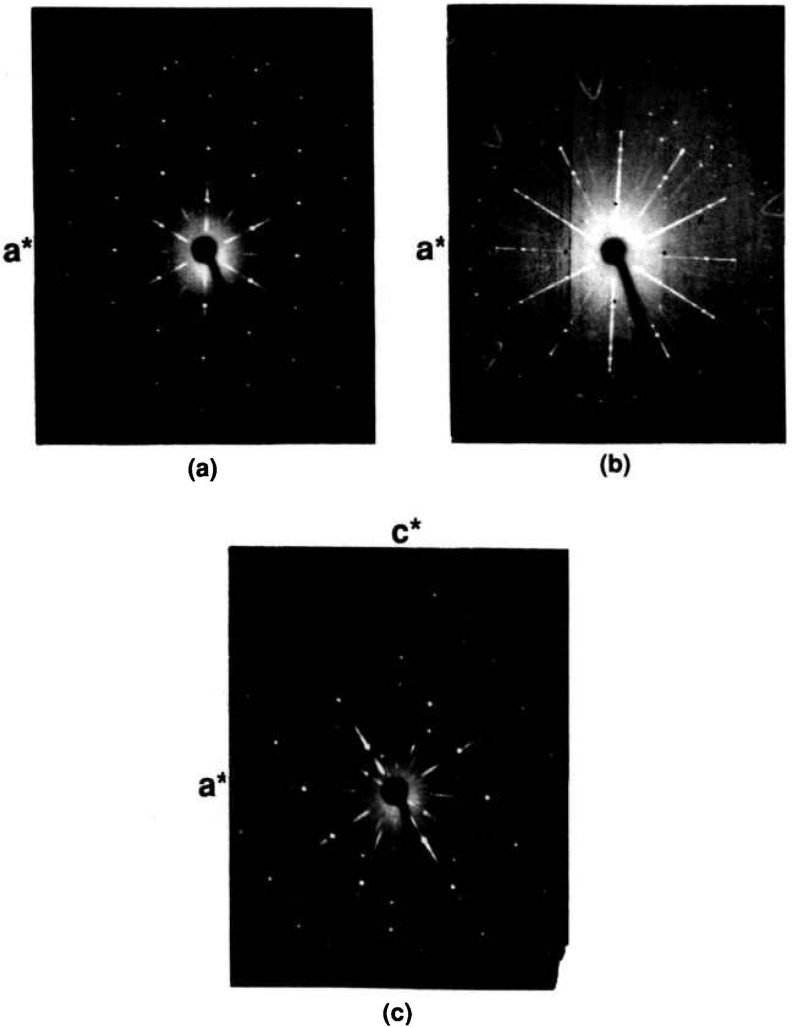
X-ray precession photographs of Sr_3_Bi_2_O_6_ (a) *hk0*, (b) unscreened *hk0* and (c) *h0l.*
